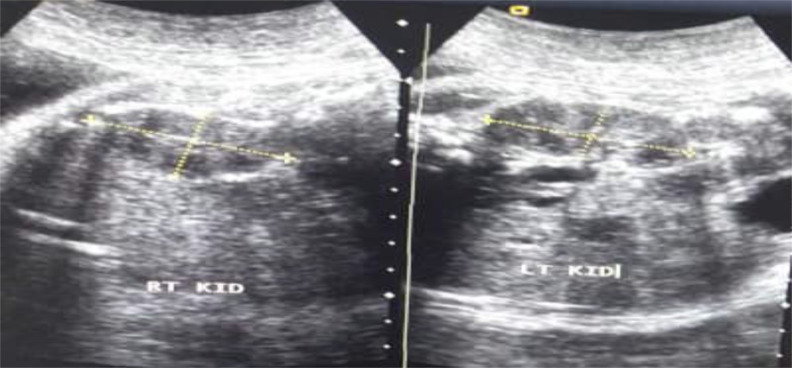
Sonogram of foetal kidney lengths and widths measurements (yellow dotted lines) at 39 weeks' gestational age; Right kidney length = 4.76 cm, Right kidney width = 2.42 cm; Left kidney length = 4.45 cm, Left kidney width = 2.20 cm
